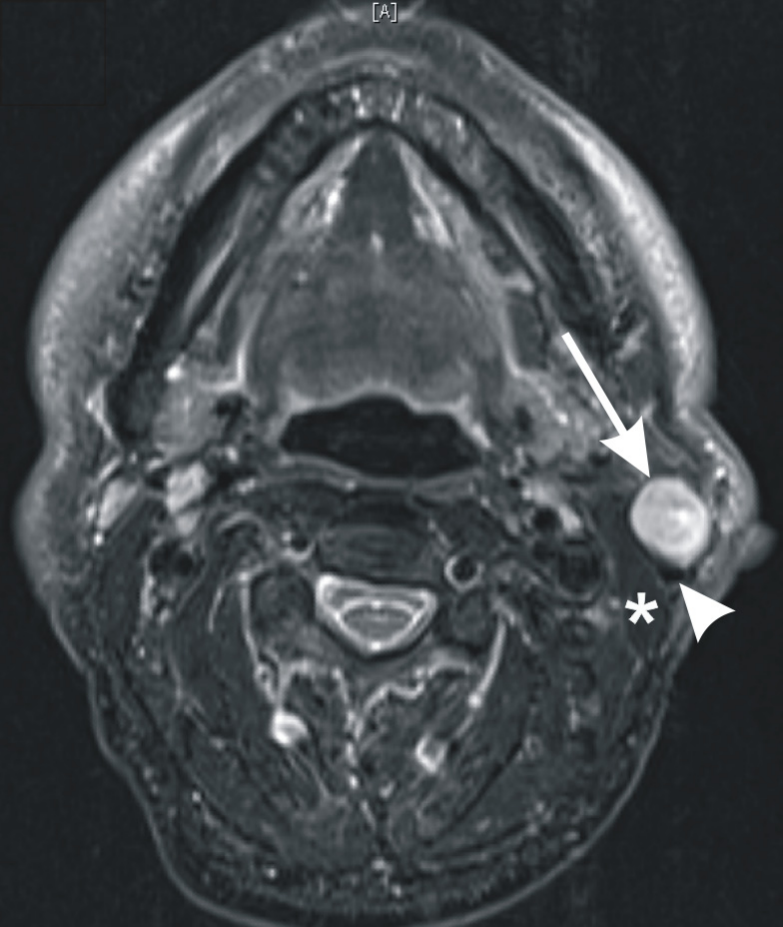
Axial MR image. (T2-weighted) of a 2 × 2 cm mass with hyperintense signal (arrow) directly bordering the lateral part of left sternocleidomastoid muscle (asterisk) and displacing the extern jugular vein (arrow head) dorsally. Cranially there was no clear demarcation to the left parotid gland.
